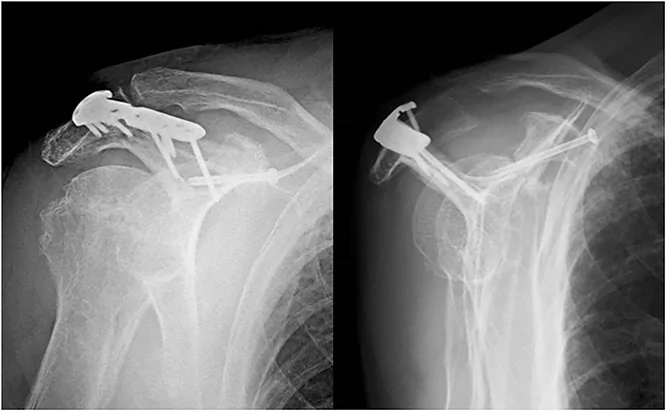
Postoperative radiographs of the right scapula obtained immediately after surgery.
